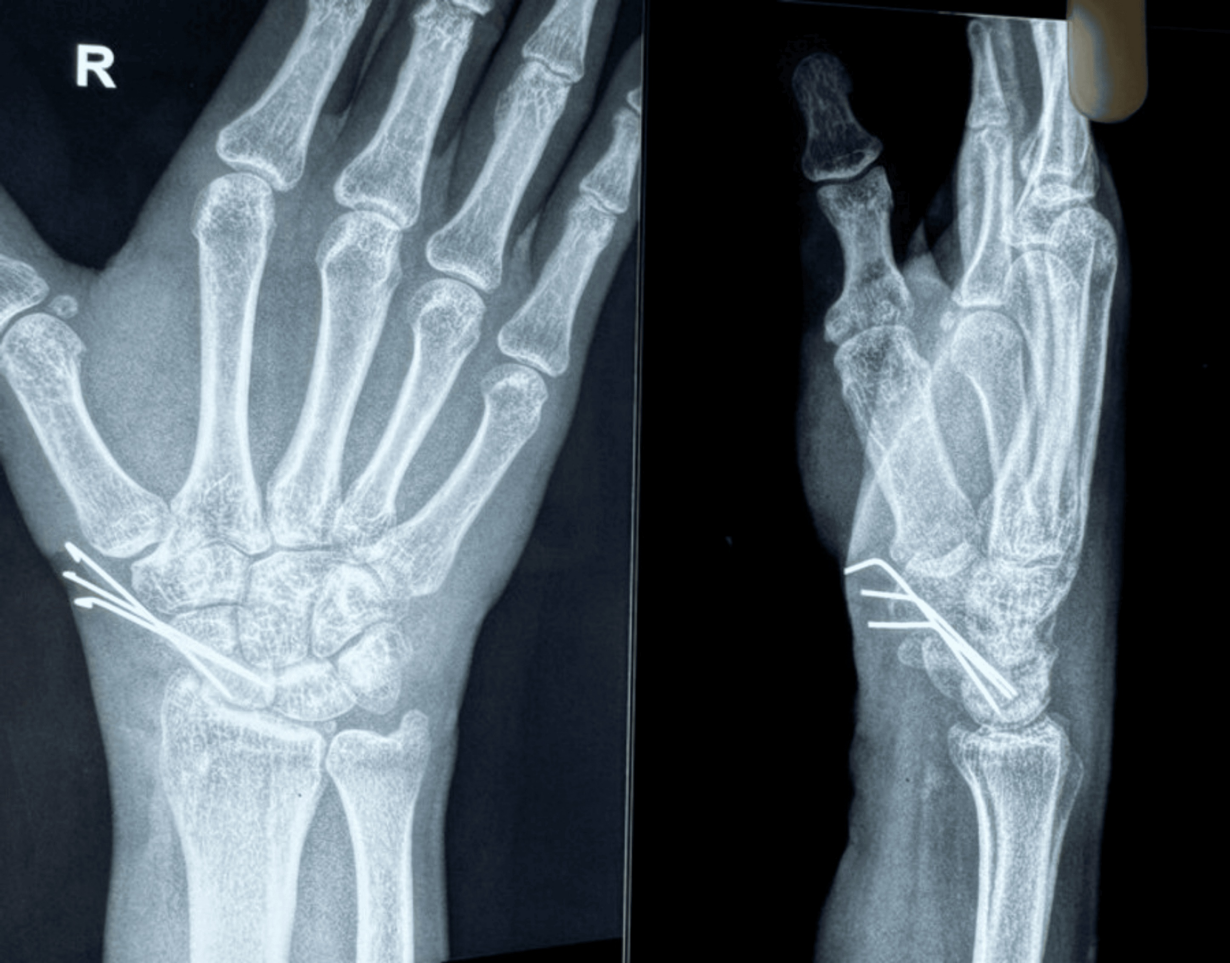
Postoperative X-ray at one month
